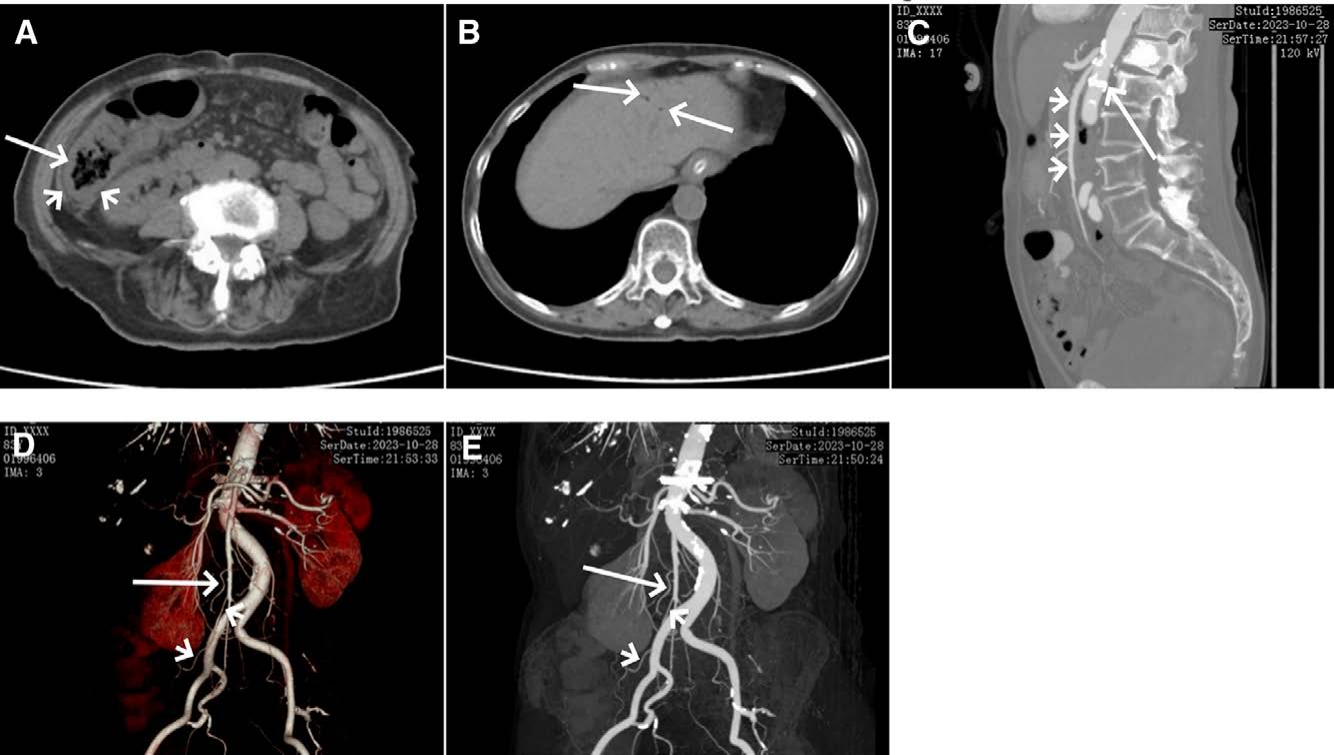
Enhanced abdominal computed tomography (CT) performed 5 hours after the sudden onset of severe abdominal pain on the 5th postoperative day. (A) Thickening of the colonic hepatic flexure wall (arrow) with disappearance of intramural gas and blurry infiltration of the surrounding fat spaces (arrowheads). (B) Substantial reduction in gas within the intrahepatic portal veins, with slight residual gas (arrows) in the left upper segment of the liver. (C) Multiple calcified plaques (arrow) visible in the abdominal aortic wall, with no filling defect in the SMA (arrowheads). (D, E) The natural course of the right colic artery (arrow) and the ileocolic artery (arrowheads) is shown, without filling defects, stenosis, or occlusion.
